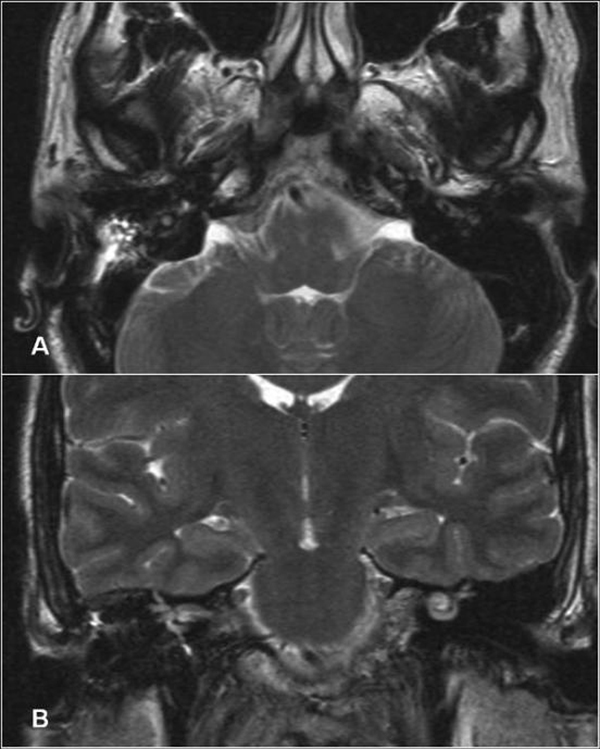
Axial (A) and coronal (B) T2-weighted magnetic resonance imaging (MRI) scan one-year post-operative excision showing no sign of recurrence.
